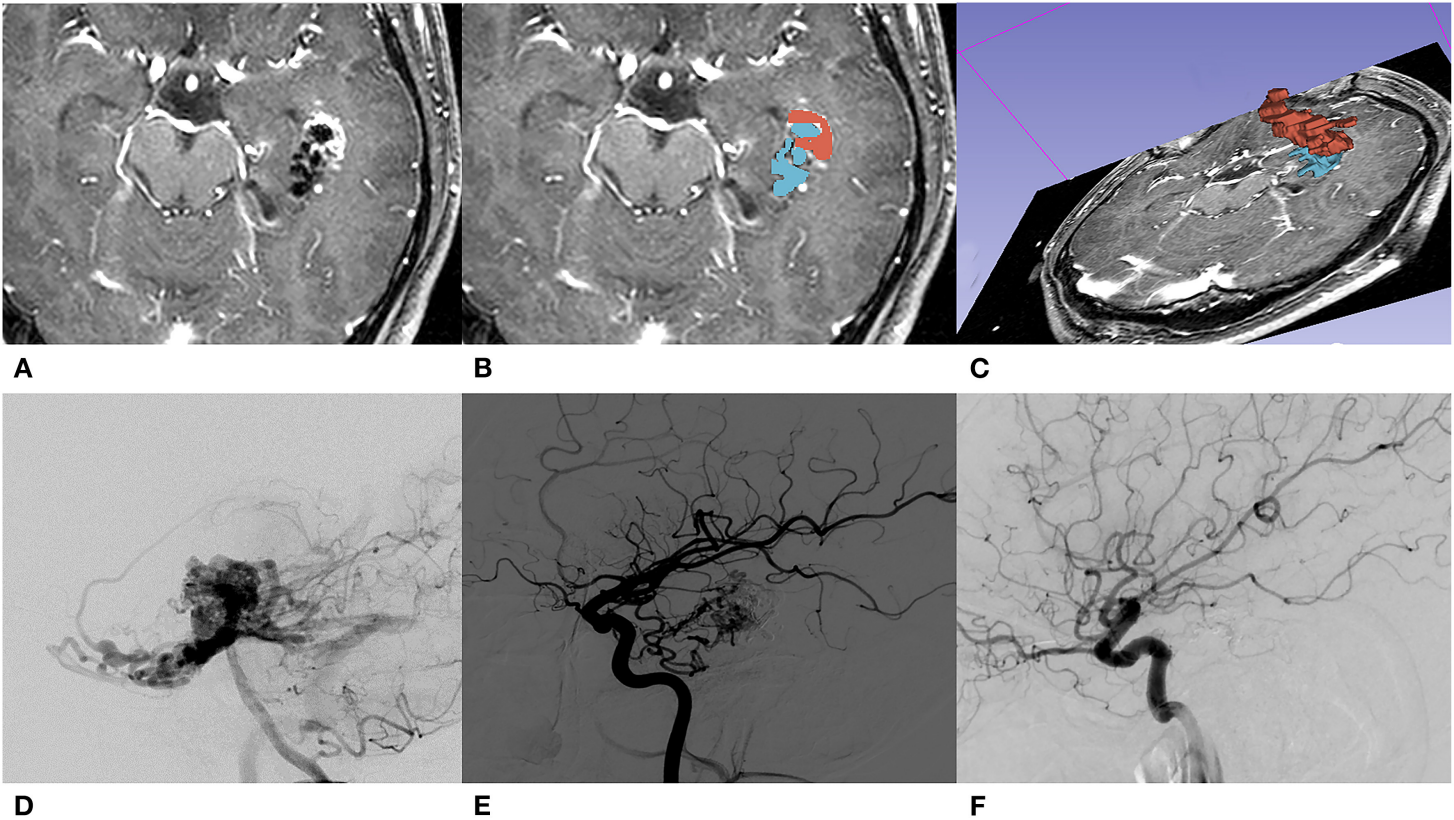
A 31-year-old male with initial presentation of epilepsy. (A) The embolized AVM located in the left temporal lobe is shown on T1 contrast-enhancement series of pre-GKS 3D-time-of-flight (TOF) MRI. (B) Segmentations of nidus (red) and embolic agent (blue) were both manually contoured slice by slice. (C) A 3D reconstruction of manually contoured region of interest (ROI) in a 3D slicer. (D) Pre-EMB angiography of the left vertebral artery; the nidus was mainly fed by the left posterior cerebral artery. (E) After embolization, branches of the left middle carotid artery feed the residual nidus. (F) DSA performed 36 months after GKS showed that the nidus was totally obliterated.
